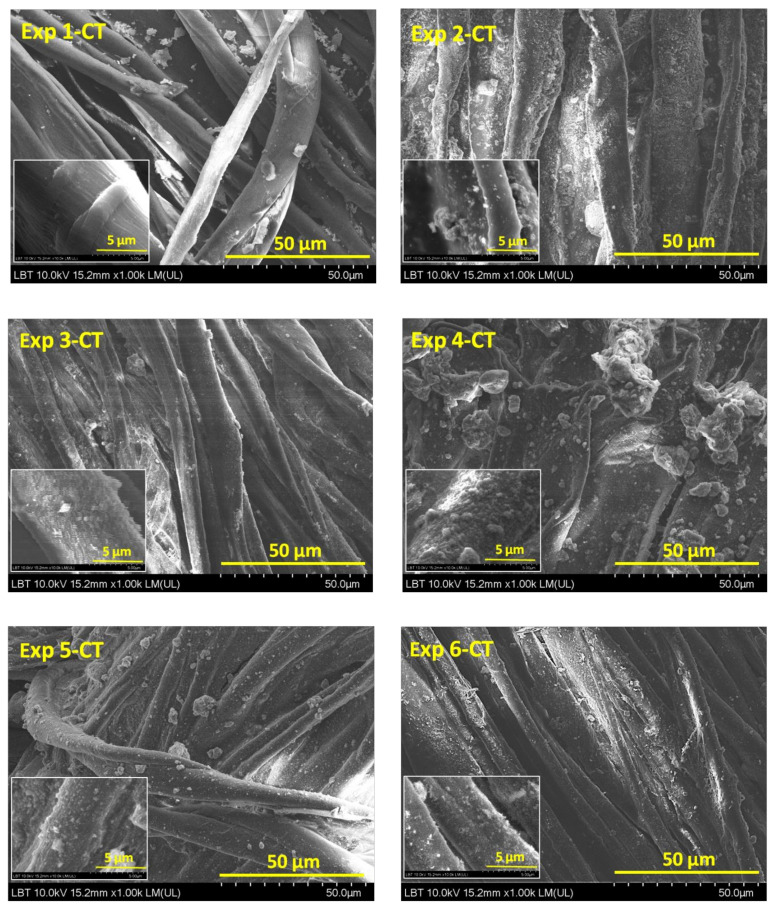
SEM images of Exp 1-CT…Exp 6-CT samples with magnification power of ×1000.
